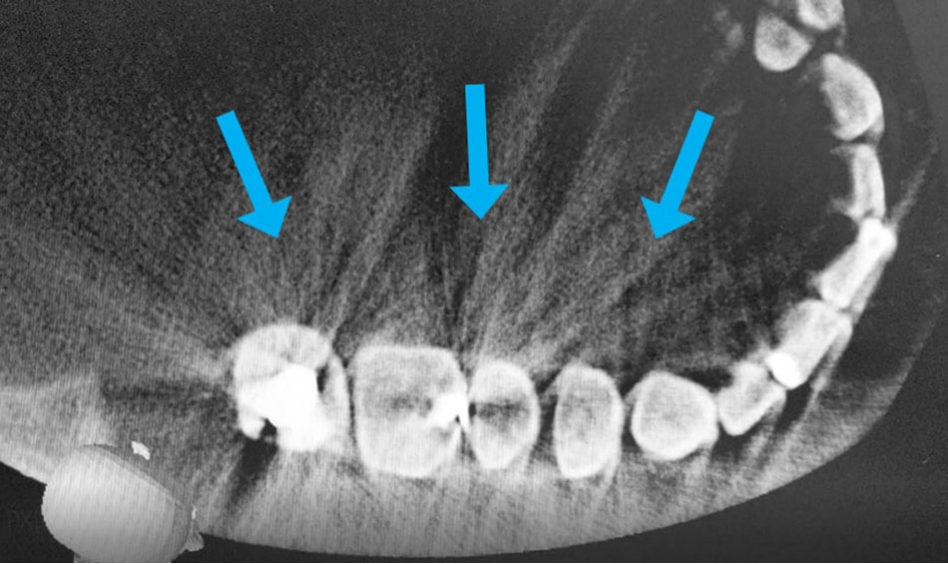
CBCT scan of teeth with amalgam restorations. Hyperdense streaks exhibited on the teeth with amalgam restorations on a CBCT scan.
